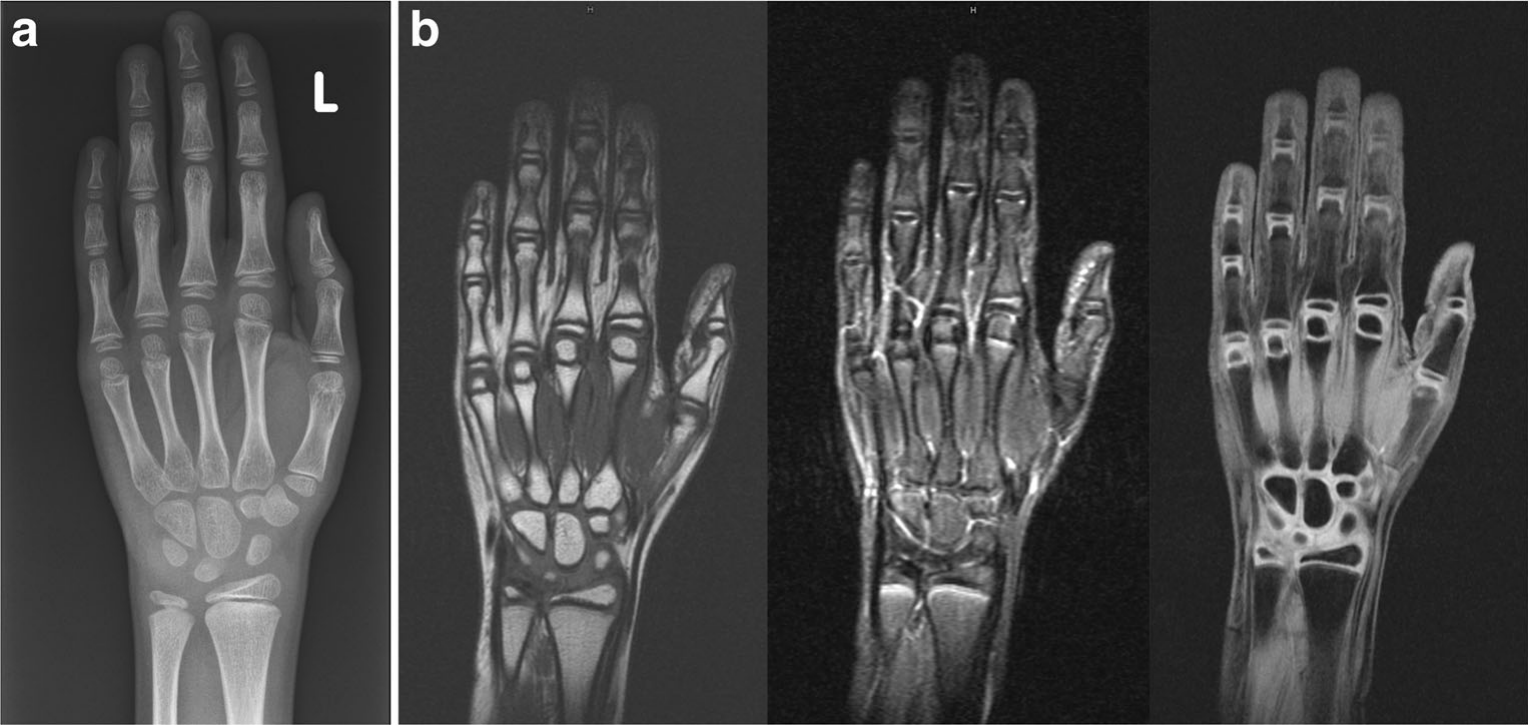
(a) Radiograph of the left hand and (b) coronal T1 SE, T1 TIRM, and T1 VIBE-3D-WE (from left to right) of an 11-year-old male with growth failure. The image quality of T1 VIBE-3D-WE was evaluated as better than the other sequences
